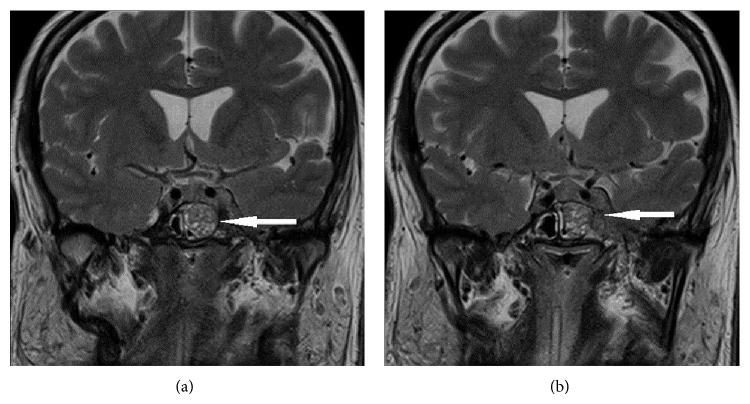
MRI brain (T2-weighted, contrast, and coronal) showing (a) pituitary involvement and (b) invasion into left cavernous sinus (indicated by arrows).
